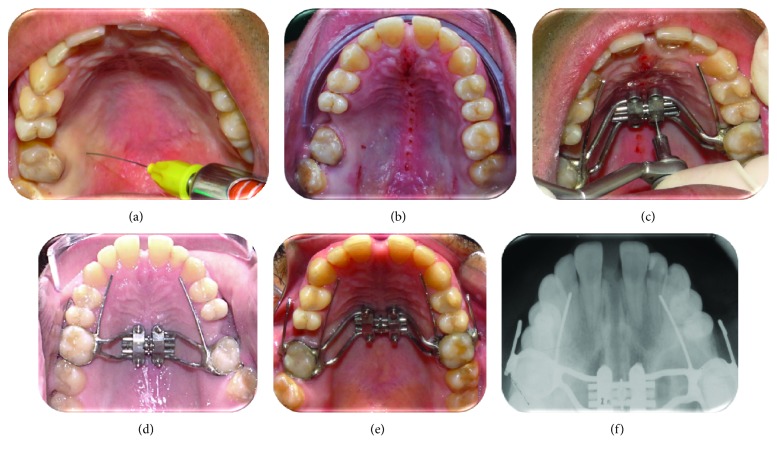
Protocol of the corticopuncture procedure suggested prior MARPE insertion in a second patient: (a) nerve block anesthesia, (b) corticopuncture procedure performed using contra-angle electric screwdriver, (c) maxillary skeletal expander in place and miniscrew insertion, (d) end of procedure, (e) result after expansion, and (f) occlusal X-ray showing midpalatal suture split.
